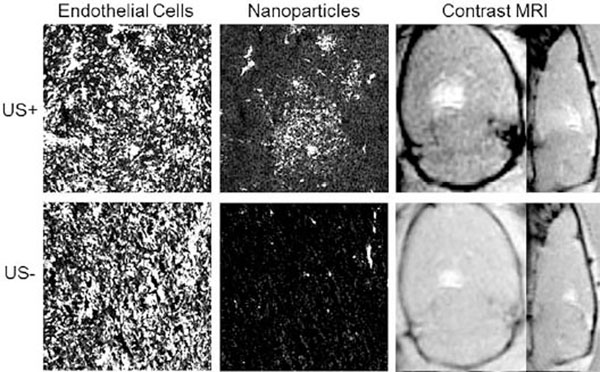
FUS and MBs are able to deliver 60 nm NPs across the BTB. Contrast-enhanced MR images demonstrate FUS-mediated BBB opening in the tumor periphery, compared to US-images. Fluorescent images show clear enhancement of NP delivery in FUS+ tumors
